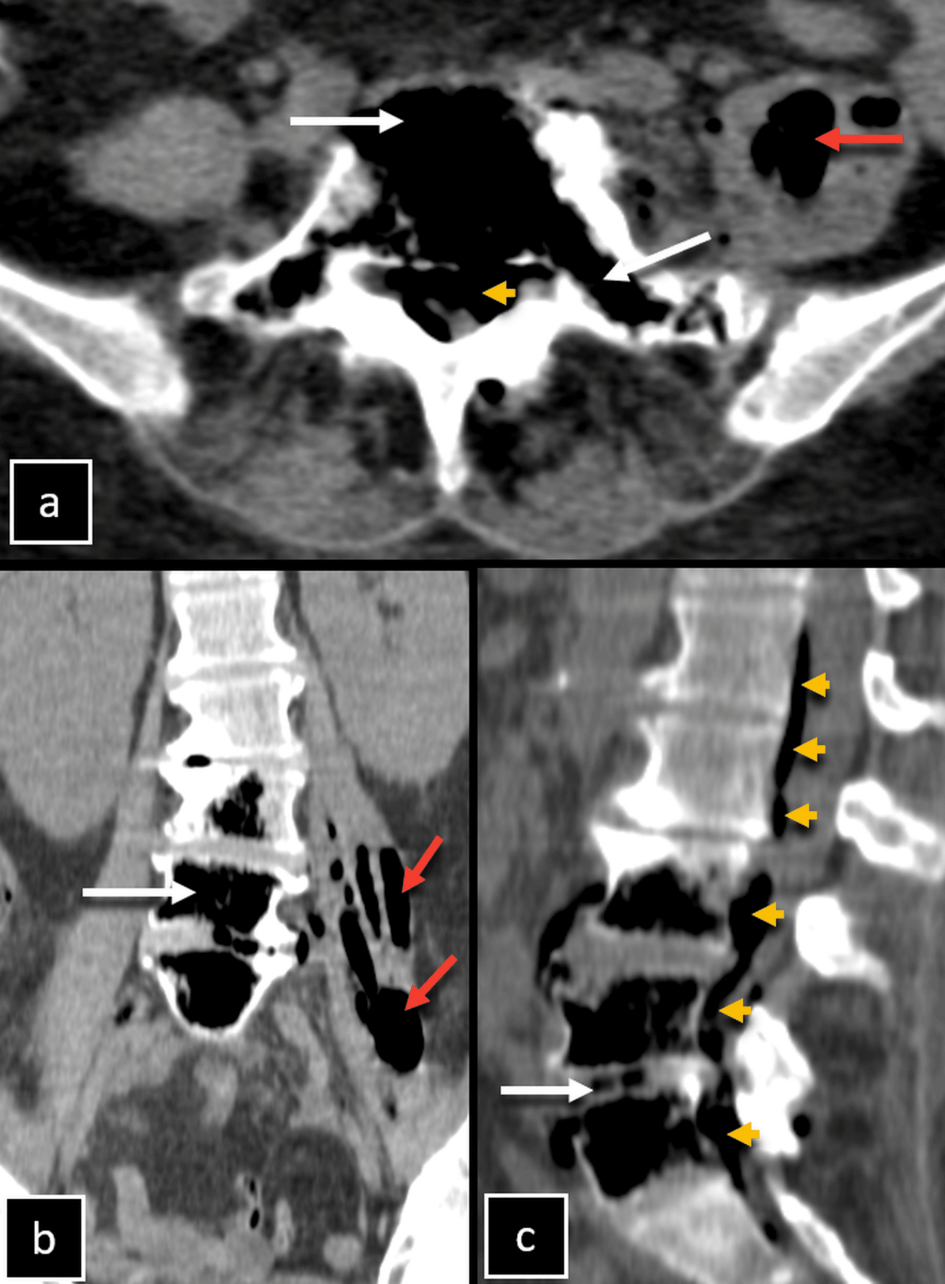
Non-contrast enhanced computed tomography (CT) of the abdomen. Non-contrast enhanced CT of the abdomen, (a) axial, (b) coronal, and (c) sagittal reformatted images show the presence of air within the vertebral bodies of L3 to L5, extending into the transverse processes and pedicle of the L5 vertebra, as well as the intervertebral discs of L2-L3 and L4-L5 (white arrows). The left psoas muscle appears bulky and heterogeneous, with multiple internal air foci (red arrow), and within the spinal canal from L1-S1 level (orange arrowheads).
